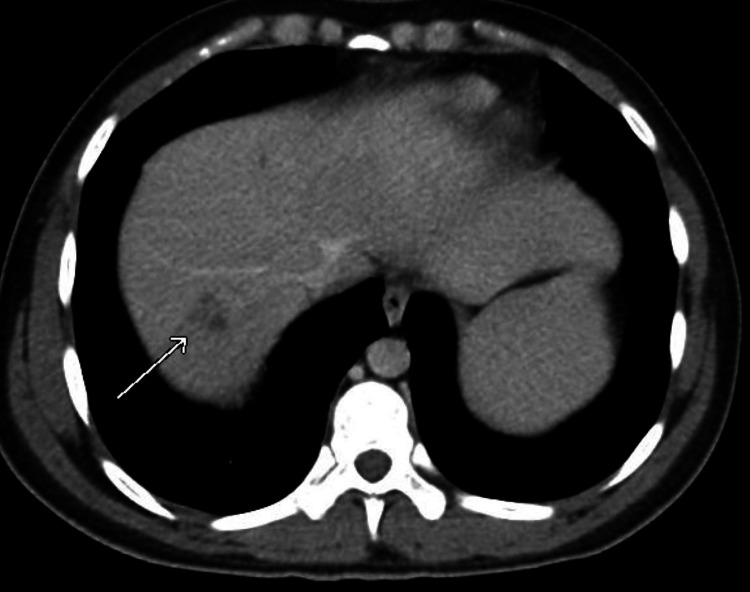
CT abdomen (axial view) showing possible liver abscess
